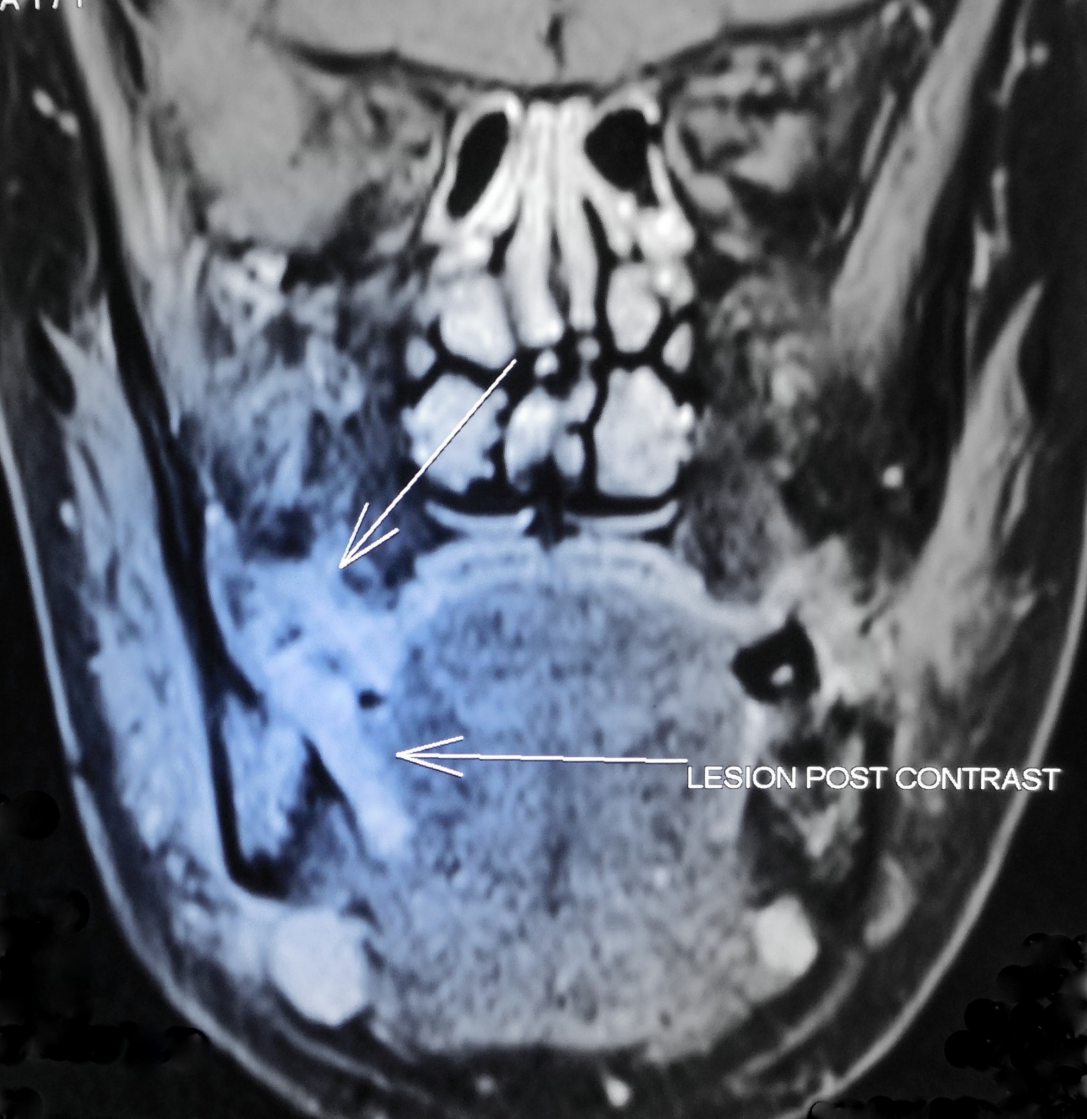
Contrast magnetic resonance imaging, coronal section showing ill-defined, irregular-shaped, well-enhancing hyperintense lesion approximately measuring 2.6 × 1.8 × 1.5 cm at right mandibular alveolar process extending into adjacent gingival mucosa, right lateral aspect of tongue and right side floor of mouth involving right mylohyoid muscle. The lesion causes erosion of posterior aspect of body of mandible. Multiple enlarged lymph nodes were noted in level I of both sides neck (largest measuring 1.6 × 1.3 cm) with no significant evidence of necrosis in enlarged lymph nodes.
